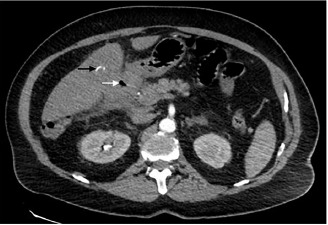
Computed tomography angiography (axial view) demonstrating a wide soft tissue tract between the gallbladder and proximal duodenum (white arrow). Calcified gallstones seen entering the proximal duodenum and along the undersurface of the right hepatic lobe (black arrow).
